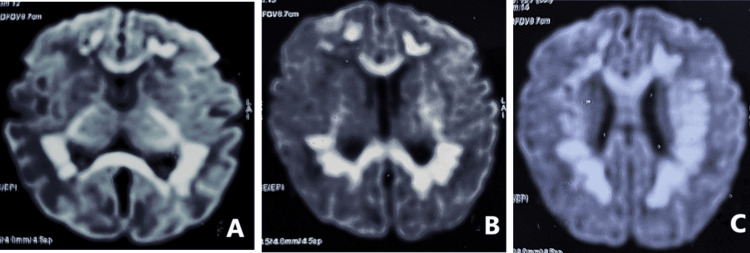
Magnetic resonance imaging demonstrating radiological hallmarks of neonatal rotavirus encephalitis (A) Symmetric diffusion restriction in the periventricular white matter, bilateral internal capsule, and thalami. These lesions appear hyperintense on diffusion-weighted imaging, consistent with cytotoxic edema. (B) Diffusion restriction is seen involving the corpus callosum and bilateral periventricular/deep white matter. (C) Diffusion restriction is seen involving the corpus callosum and bilateral periventricular/deep white matter
